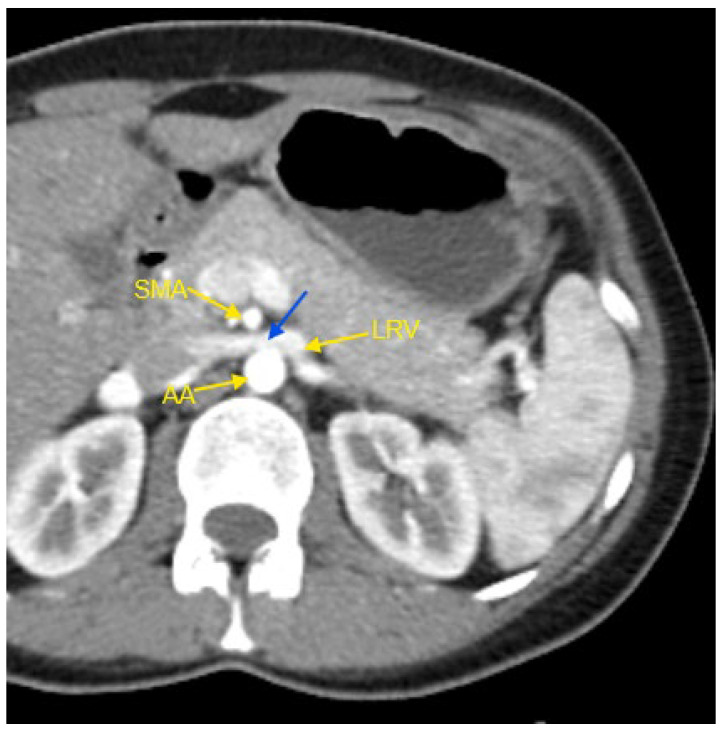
Contrast-enhanced CT imaging of a 44-year-old female patient with high blood pressure refractory to treatment, revealed characteristic findings consistent with classic Nutcracker syndrome. In the presented image, the abdominal aorta, the superior mesenteric artery, and the left renal vein are indicated by yellow arrows. The compressed segment of the left renal vein is highlighted by a blue arrow. AA—abdominal aorta; CT—computed tomography; LRV—left renal vein; SMA—superior mesenteric artery.
